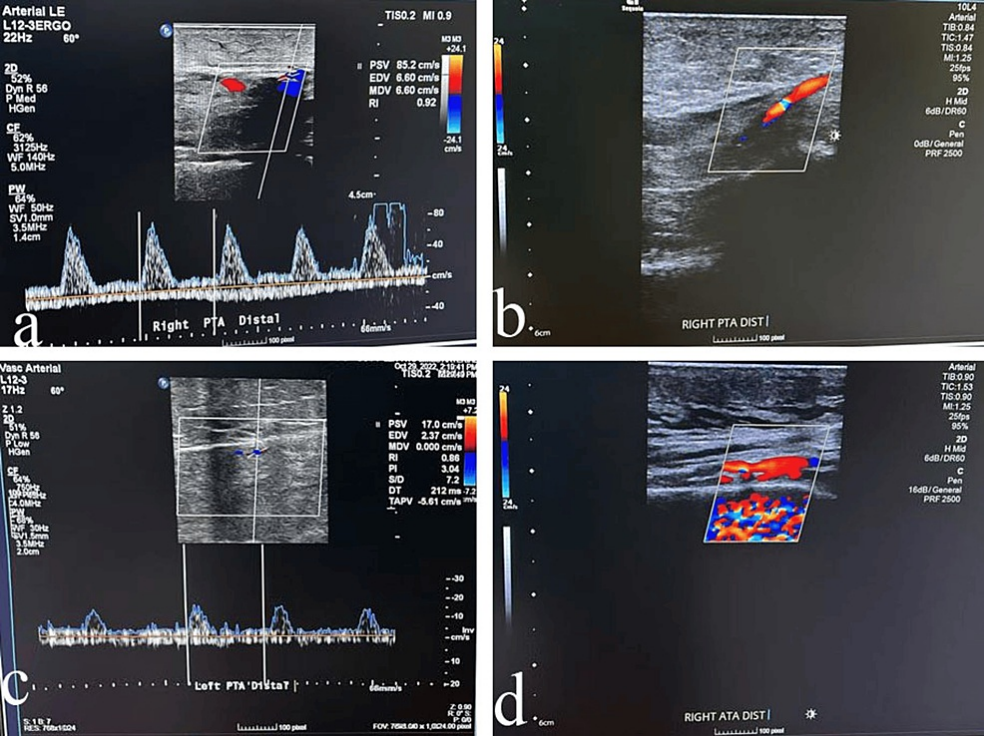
Triplex Doppler sonogram of some studied cases a- The right posterior tibial artery has been attenuated by atherosclerosis with triphasic to biphasic waveforms and no definite segment of occlusion; b- The right posterior tibial artery is attenuated by atherosclerosis without definite obstruction; c- The left posterior tibial artery is patent with segmental attenuation. There are triphasic to biphasic waveforms; d- The left anterior tibial artery is attenuated by atherosclerosis without definite obstruction.
